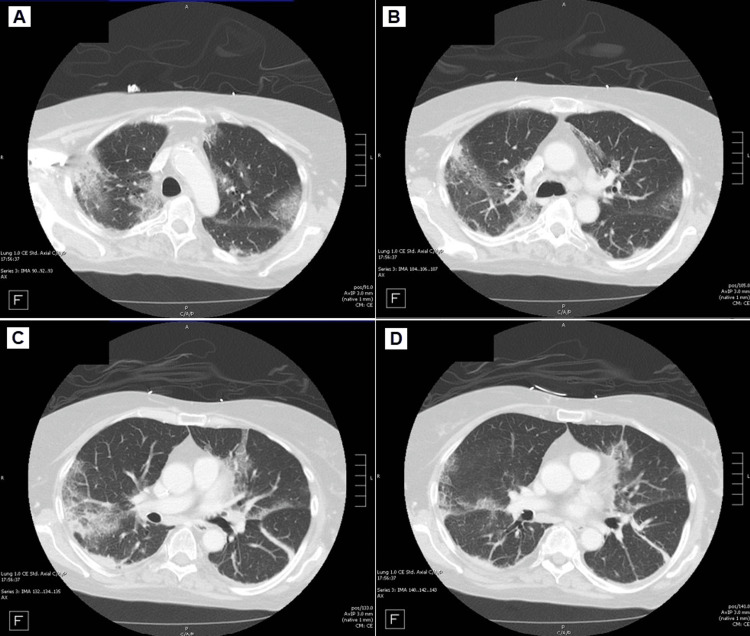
(A-D): Four panels of a CT scan of the chest on day five of admission showing upper lobe predominant interstitial thickening with ground-glass changes. CT: computed tomography
